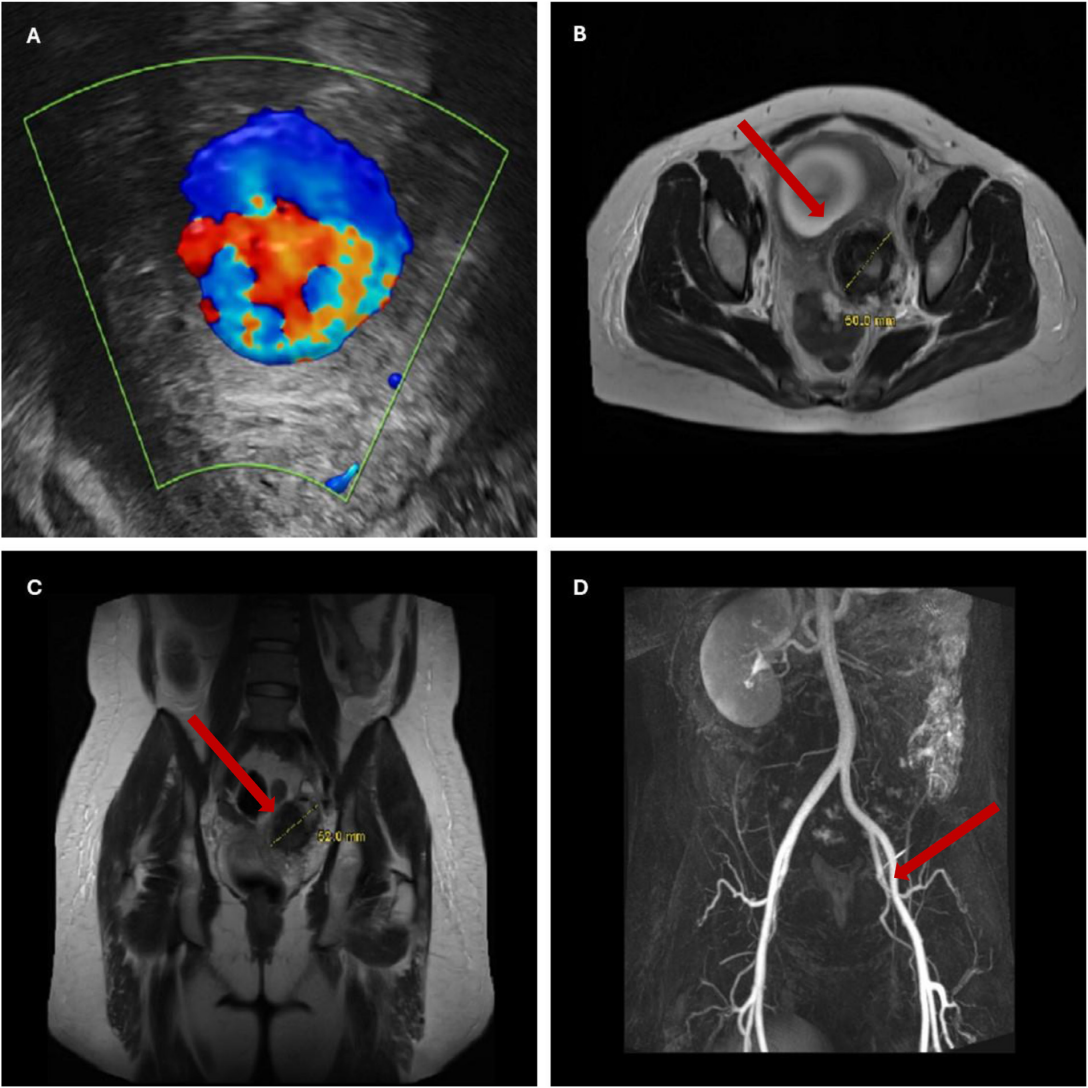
Diagnostics of the UAP. (A) The pathognomonic yin-yang sign showing the blood turbulence within the UAP of the left uterine artery in Doppler ultrasonography (GE Voluson E10 System, GE Healthcare, Zipf, Austria). (B) T2-weighted axial MRI showing a well-defined UAP. (C) T2-weighted coronal MRI showing the UAP. (D) Magnetic resonance angiography of the thrombosed UAP.
